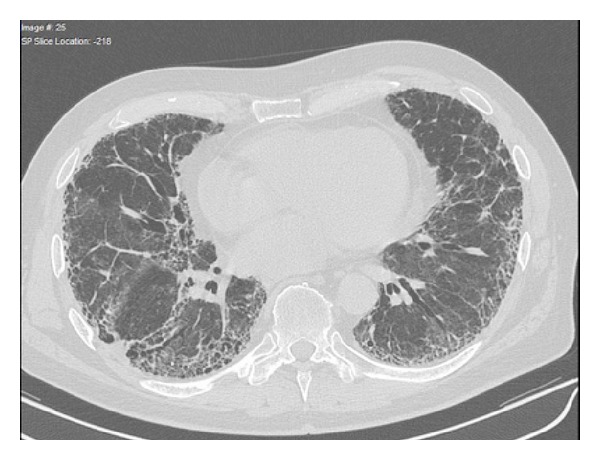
HR-CT-scan of the thorax revealed pronounced signs of pulmonary fibrosis including consolidations and honeycombing especially in basal areas.
